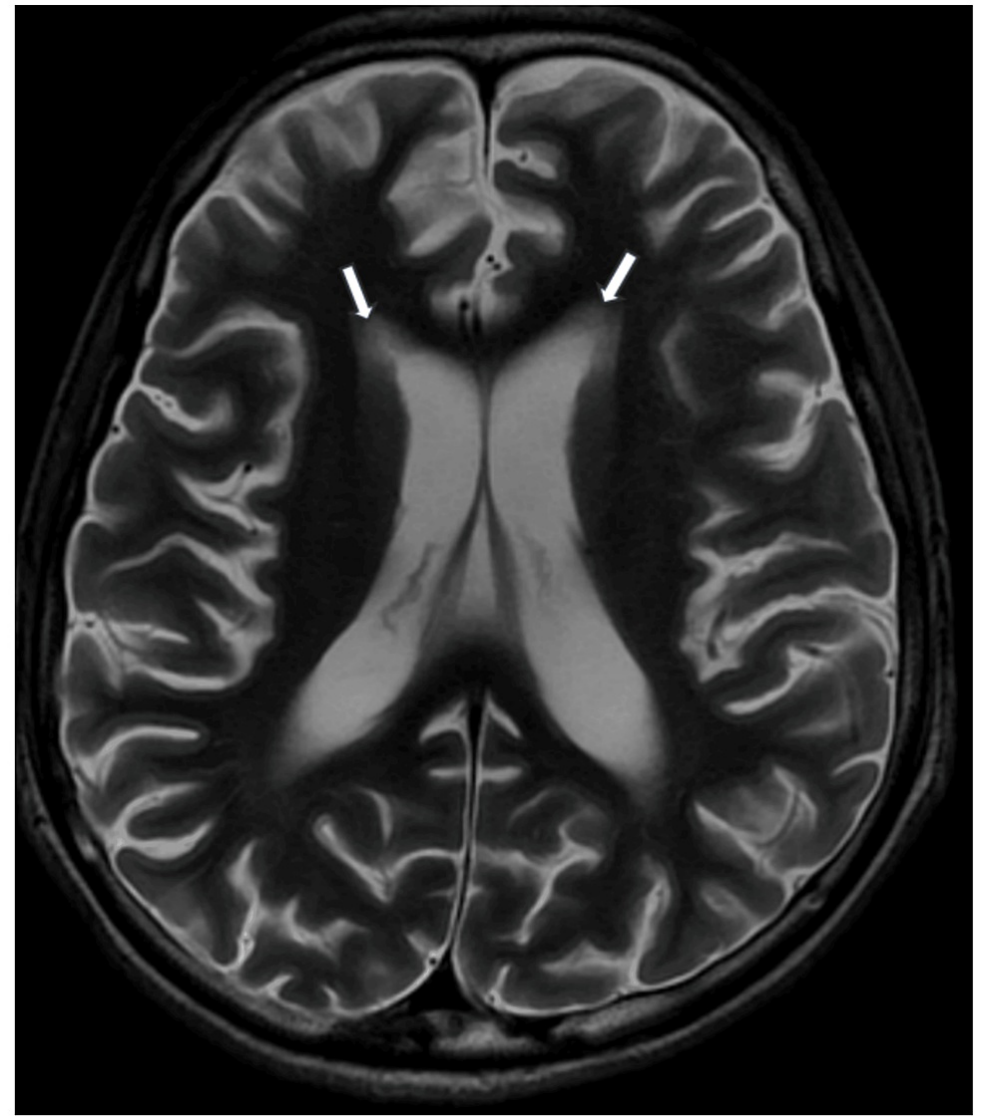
T2-weighted MRI brain (axial section) reveals diffuse periventricular white matter hyperintensities (white arrow) MRI: magnetic resonance imaging
